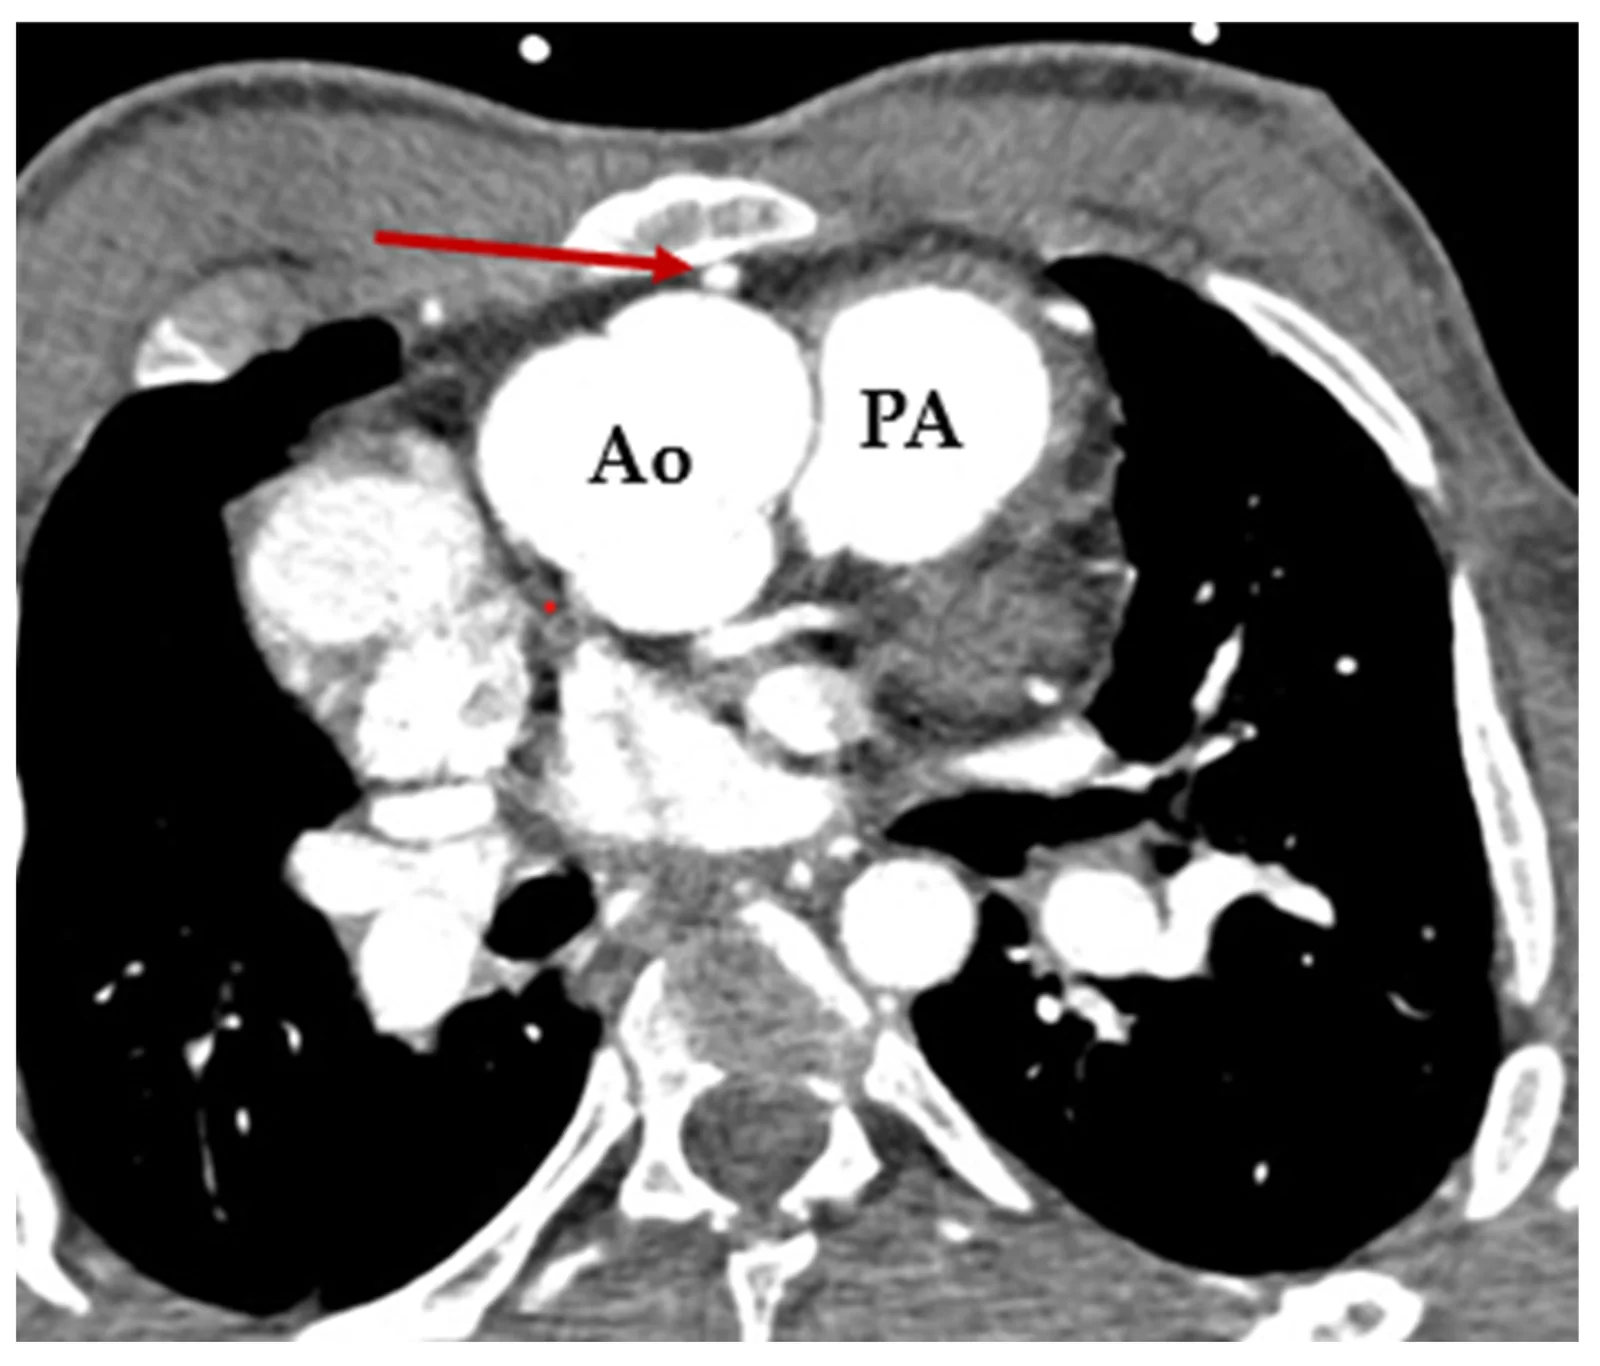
Computed tomography scan showing the dilated aortic root with the more cephalad and anteriorly displaced right coronary artery button (red arrow) in a patient who underwent Ross procedure. This can constitute a challenge during reoperation; Ao: aorta; PA: pulmonary artery.
